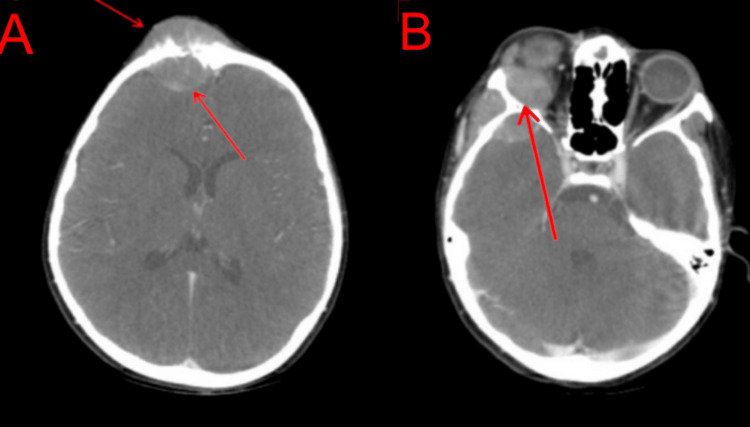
A: Contrast-enhanced CT showing enhancing soft tissue density mass involving the right half of the frontal bone with strong periosteal reaction and intracranial extension with effacement of the right frontal lobe. B: Lesion involving the right orbit and the right zygoma.
